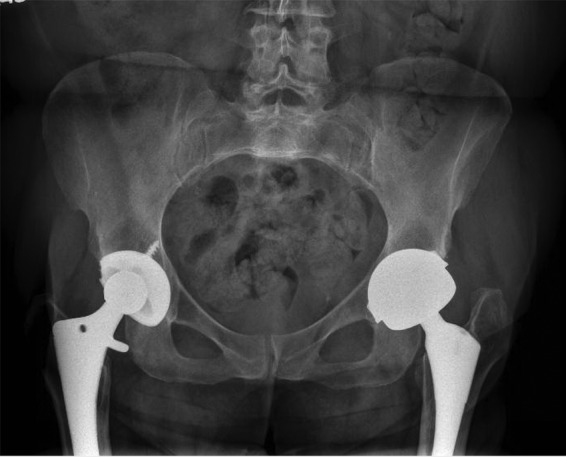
AP pelvis radiographs show right metal-on-polyethylene THA and left metal-on-metal THA. Components appear in appropriate positions without obvious signs of loosening. AP, anterior-posterior; THA, total hip arthroplasty.
